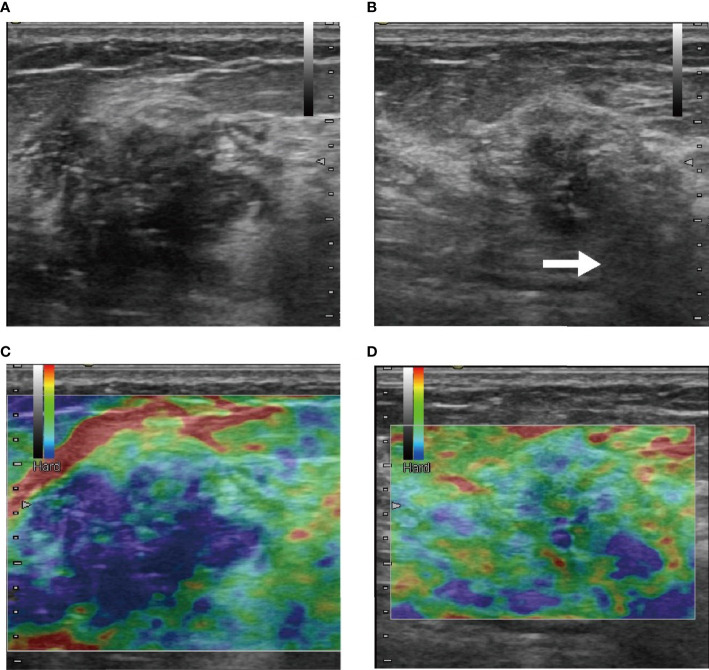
These were B-mode (A, B) and elasticity ultrasound (C, D) images of a patient who was treated with NAC and who eventually achieved pCR. (A, C) The initial images before NAC. (B, D) Images after two-cycle response. In B-mode images (A, B), size shrinkage was obvious, and the posterior acoustic changed to shadowing (white arrow) after the two-cycle response was visible. From the elasticity ultrasound images (C, D), we observed that the range of rendering blue was reduced, which mean that the elasticity score was reduced.
